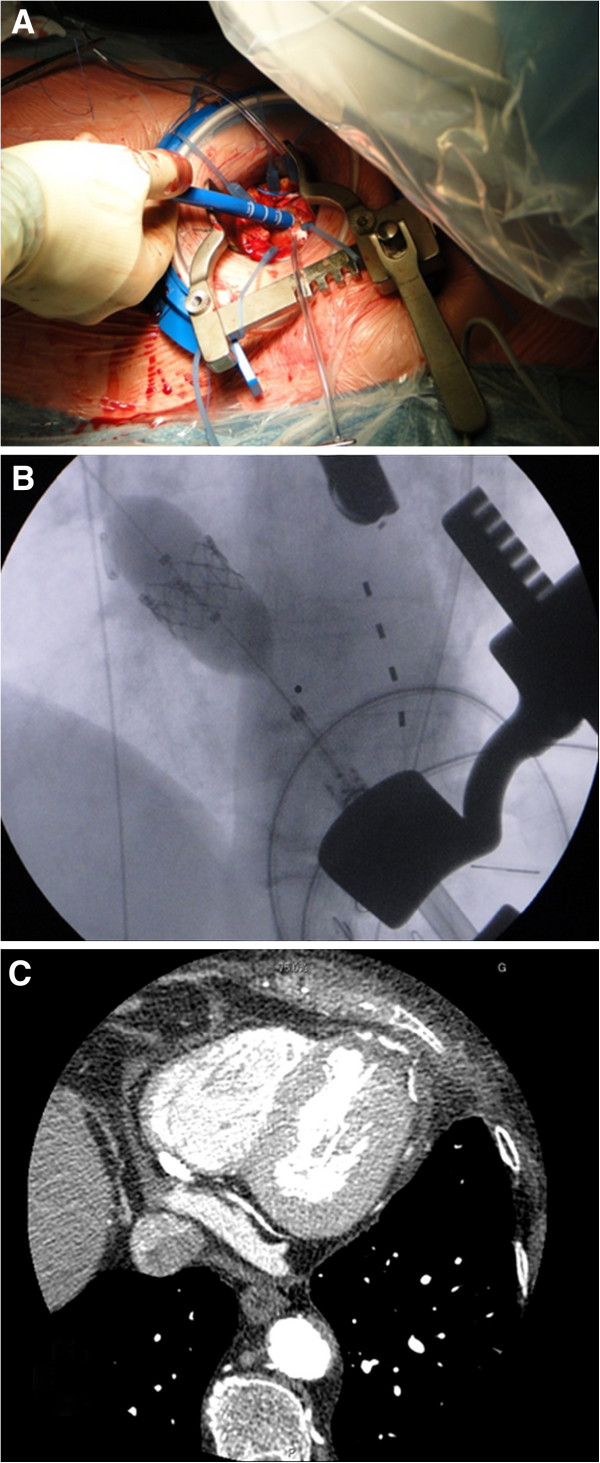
A) Intraoperative view showing the introduction of the delivery system (Ascendra™ 2) in the apex. B) Fluoroscopic view of the stent-valve deployment under rapid pacing. C) Postoperative computed tomography scan showing the good result with partial exclusion of the diverticulum.
